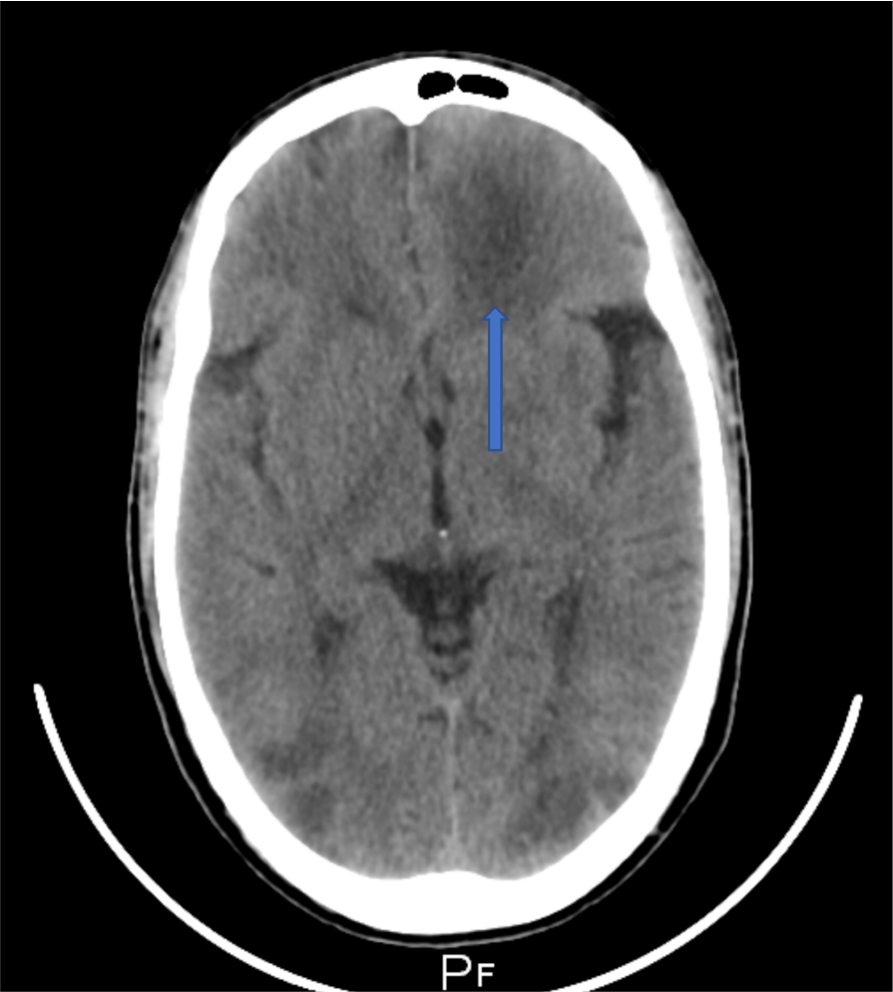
Non-contrast CT scan of the brain revealing further enlargement of the frontal hypodensity
